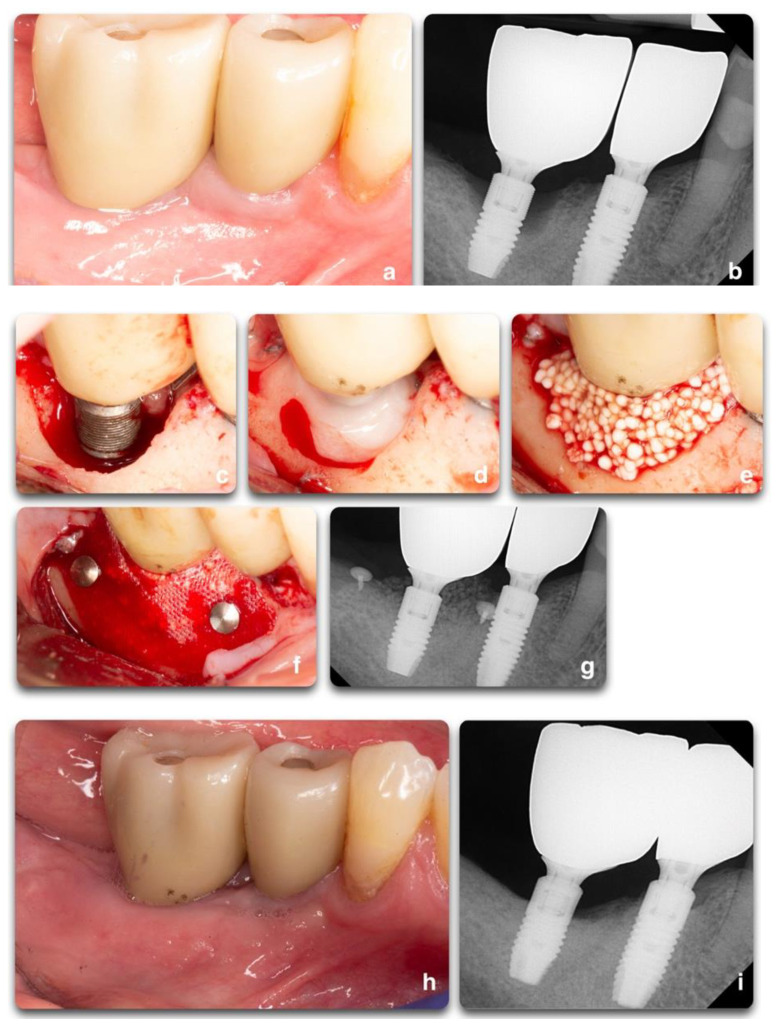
Regenerative peri-implant therapy for implant area #31. (a,b) Pre-operative clinical and radiographic presentation. BoP, SUP, PD 10mm. (c) Defect configuration after the elevation of the full-thickness flap on implant #31. (d) Implant surface decontamination with rotating titanium brushes, saline and chlorhexidine rinse, and the application of PrefGel^®^ 24% EDTA (2021 Institut Straumann AG^®^). (e,f) Bone grafting application (GUIDOR^®^ easy-graft^®^ CLASSIC Alloplastic Bone Grafting System © © Copyright Collagen Matrix, Inc.—Allendale, NJ, USA), covered by the GUIDOR^®^ matrix barrier membrane, and stabilized by two titanium tacks. (g) Radiograph showing the grafting material at the time of surgery completion. (h,i) 13 months post-operative clinical and radiographic outcomes. Partial fill of the intra-bony defect with a residual supra-crestal defect.
